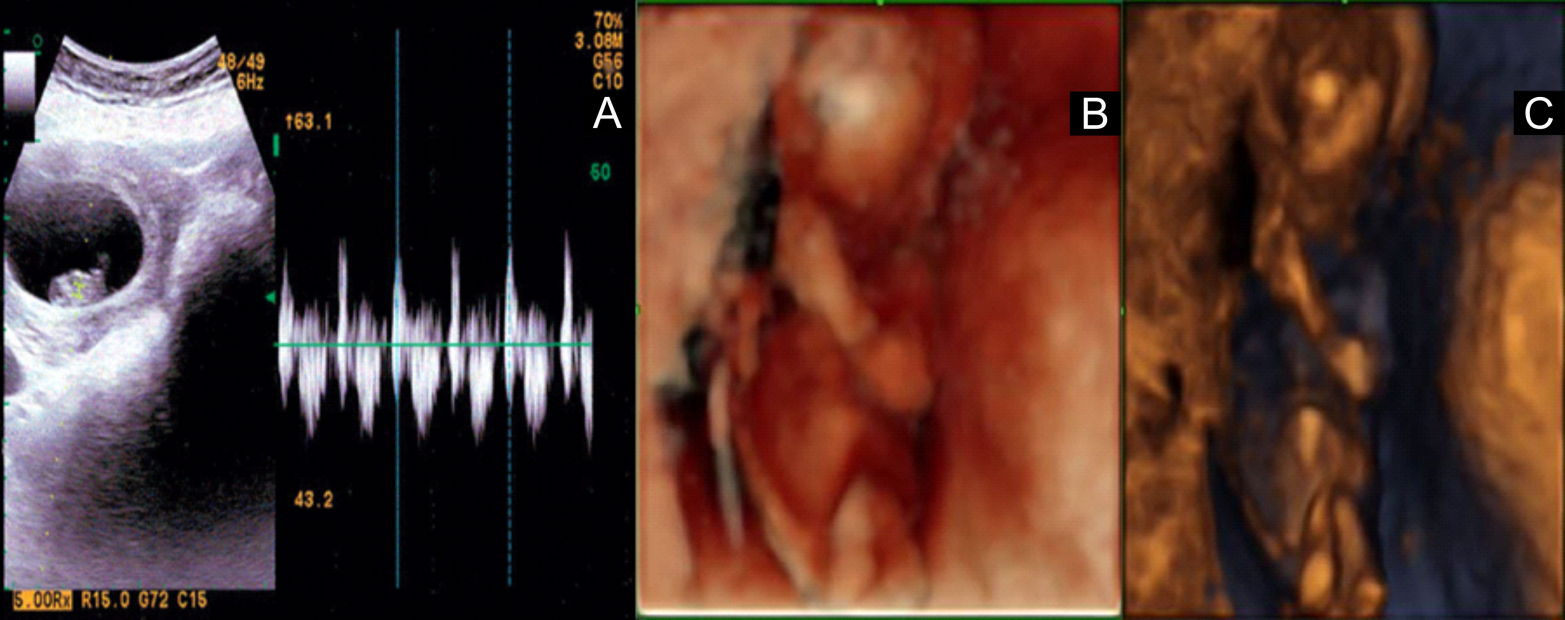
(A) First ultrasound at 10.5 weeks of gestation without alterations. (B, C) Normal structural ultrasound at 15.4 weeks of gestation (according to the last menstrual period). Average fetometry of 15.5 weeks for a weight of 124 g.
